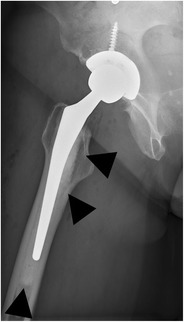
AP radiograph. Cemented total hip arthroplasty, single acetabular screw fixation. In a hybrid cemented arthroplasty, the femoral stem is fixed with cement (*arrowheads*)
